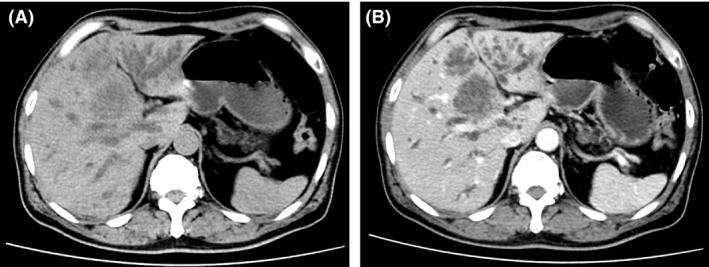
Mass‐forming cholangiocarcinoma. The axial unenhanced abdominal computed tomography image shows two ill‐defined low‐density masses at right lobe of the liver with moderately dilated intrahepatic ducts (A), and these masses show minimal peripheral enhancement in the arterial phase (B).
